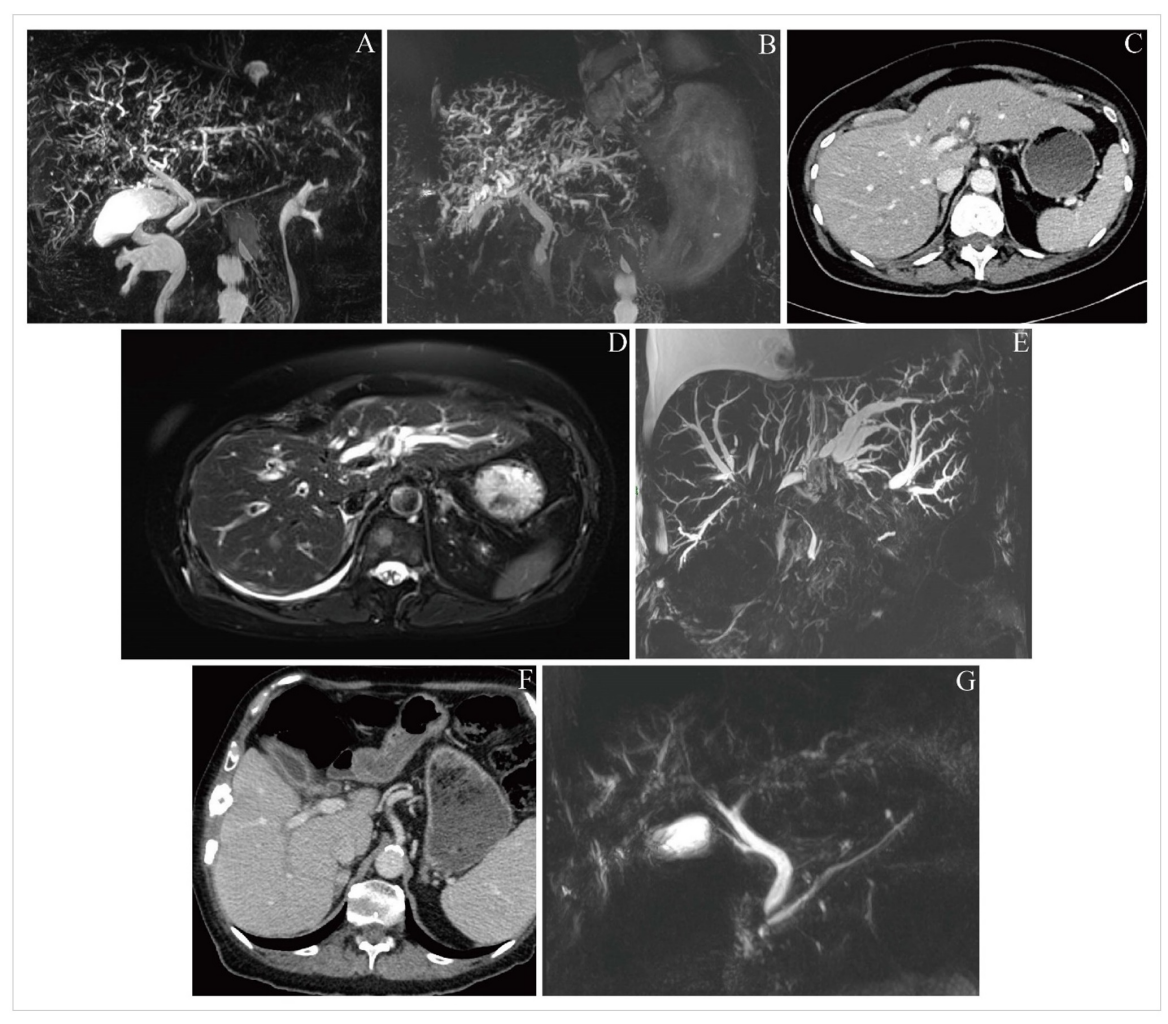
Representative imaging findings for included patients. (A) Case 1; cholangiographic image from magnetic resonance cholangiopancreatography (MRCP) on day 76 after diagnosis of immune checkpoint inhibitor-induced hepatotoxicity (ICI-H), demonstrating intrahepatic duct abnormalities consistent with sclerosing cholangitis; (B) case 1; cholangiographic image from MRCP on day 513, showing stable intrahepatic duct dilatation compared with prior imaging; (C) case 2; computed tomography (CT) image on day 206 after ICI-H diagnosis, demonstrating mild biliary dilatation; (D) case 2; T2 MR image on day 210, demonstrating more pronounced features of sclerosing cholangitis compared with Figure 2C; (E) case 2; cholangiographic image from MRCP on day 217, clearly demonstrating marked intrahepatic biliary dilatation, and a long segment of stenosis within the common bile duct (CBD); (F) case 3; CT image from 15 days before ICI-H diagnosis, demonstrating enhancement of the gallbladder, central bile ducts and CBD; (G) case 3; cholangiographic image from MRCP on day 26 after ICI-H diagnosis, demonstrating the same abnormalities as seen in Figure 2E, as well as intrahepatic duct abnormalities
